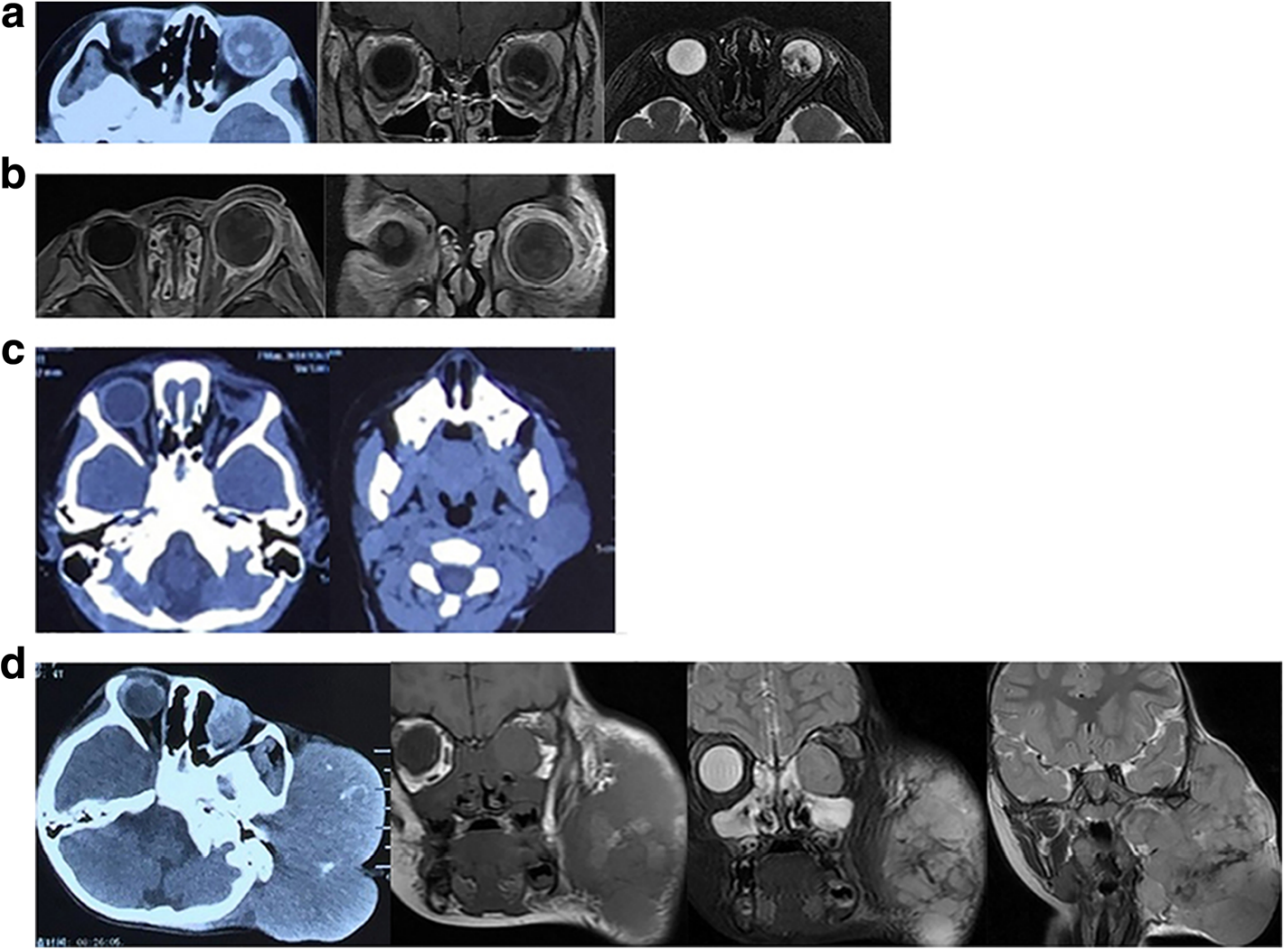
a: (Dec. 2012) Axial orbital CT scan shows a heterogeneous intraconal mass lesion with small foci of hyperdensity. MRI T1 scan shows a hyperintense lesion in the posterior part of the left globe that is hypointense in T2 MRI image. b: (Nov. 2013) MRI scan showing the larger left eye and mass outside of the left orbit. (Compare with MRI scan in Fig. 2a). c: (May 2014) CT scans show tumour regression in the left orbit and a small moderately intense mass on the left side of the neck with extension to the parotid and submandibular glands. d: (Dec. 2014) Axial CT scans show attenuation to muscle and an ill-defined solitary mass in the left orbit as well as a very large and moderately intense mass on the left side of the neck with extension to the parotid and submandibular glands. MRI scan shows iso-intensity on T1-weighted images with moderate to marked enhancement post-contrast with fat suppression, hyper-intensity on T2-weighted images in the left orbit and a large mass that was iso-intense on T1-weighted images with moderate to marked enhancement
